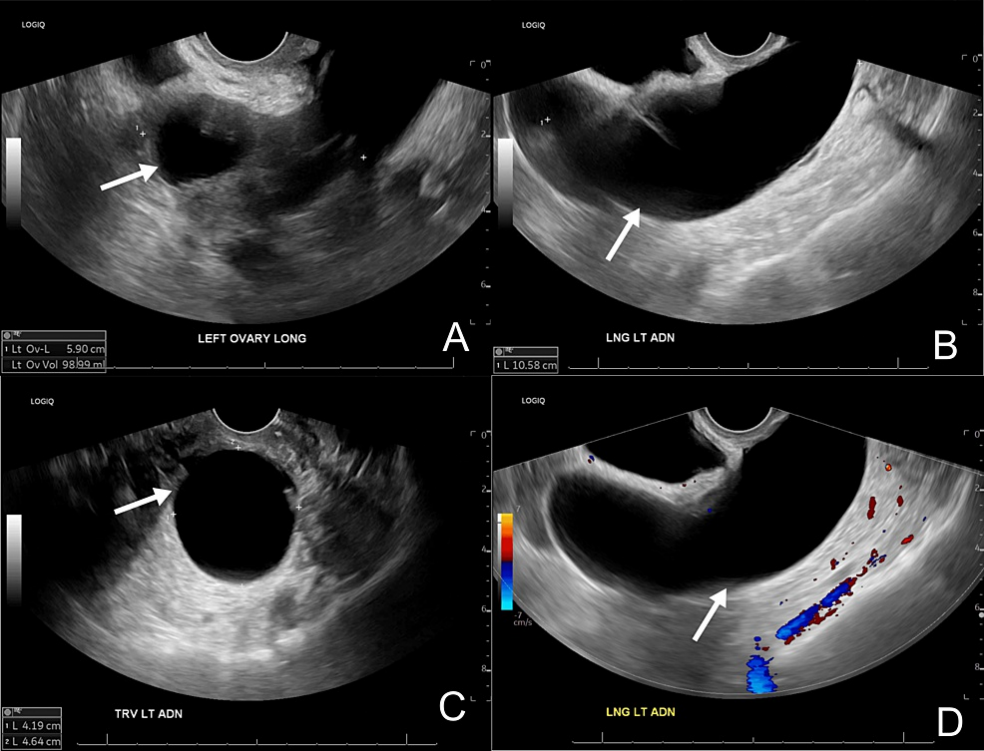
Transvaginal ultrasound Transvaginal ultrasound shows (A) the left ovary in the long axis that is attached to (B) a tubular-shaped, anechoic cyst. (C) Transverse view of the tubular-shaped cyst. (D) Doppler study shows an avascular tubular mass.
